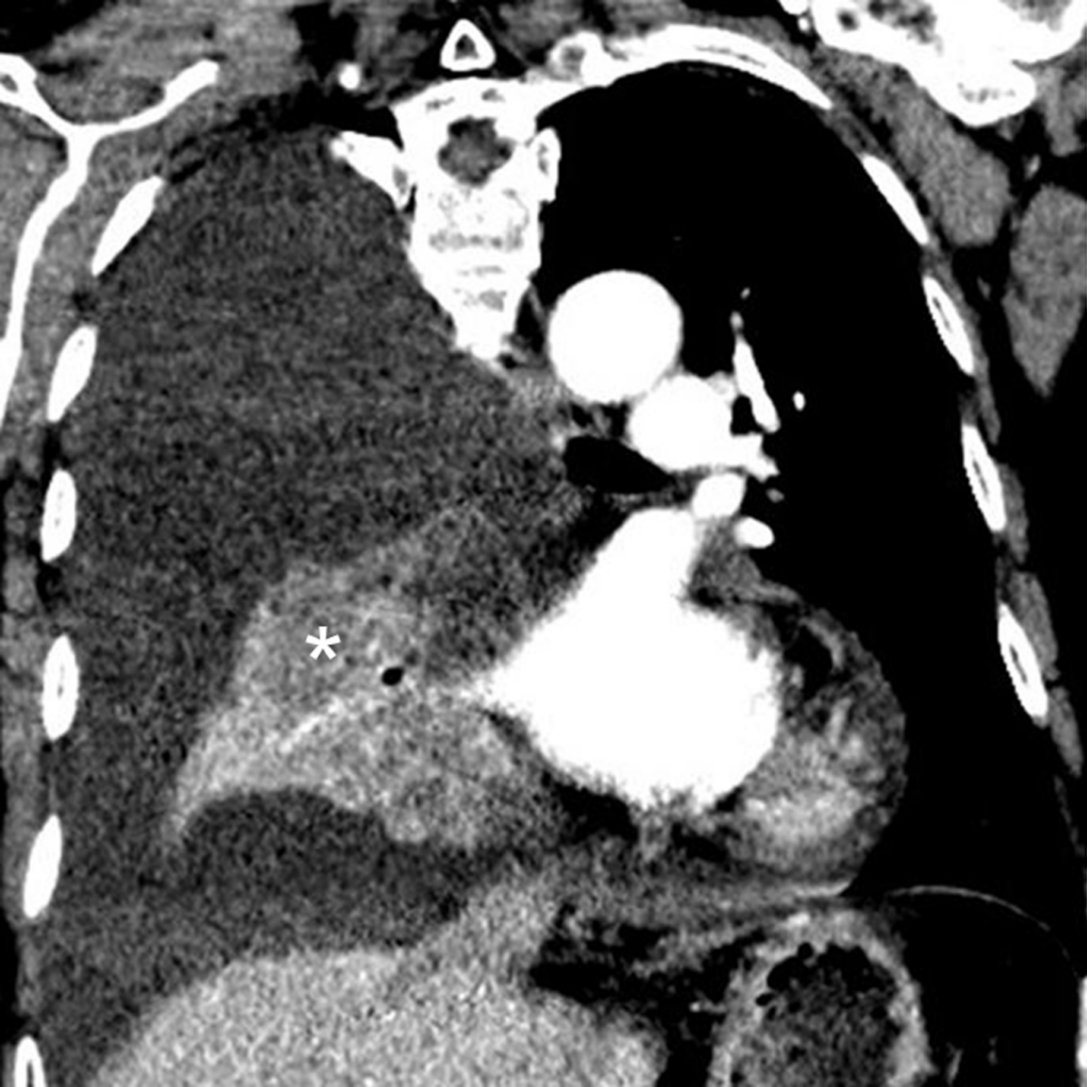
Computed tomography (CT) of the chest confirms a large right pleural effusion with collapse of the middle and lower lobe. The bulging nodular hypoenhancing mass (*) in the right lower lobe is suspicious for primary bronchogenic malignancy.
